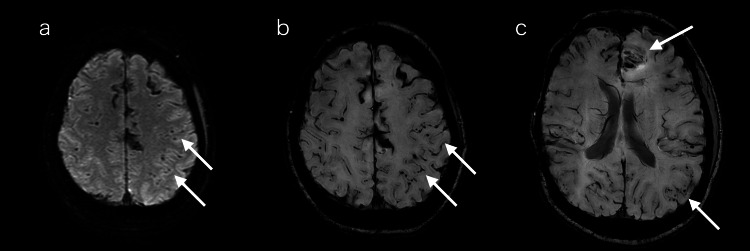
Brain MRI images on day one T2-weighted imaging showed multiple superficial hemosiderin depositions; white arrows indicated micro-bleedings. (a) Susceptibility-weighted imaging showed multilobed micro- and macro-hemorrhages and extensive superficial siderosis; (b, c) White arrows indicated hemorrhages and superficial siderosis.
